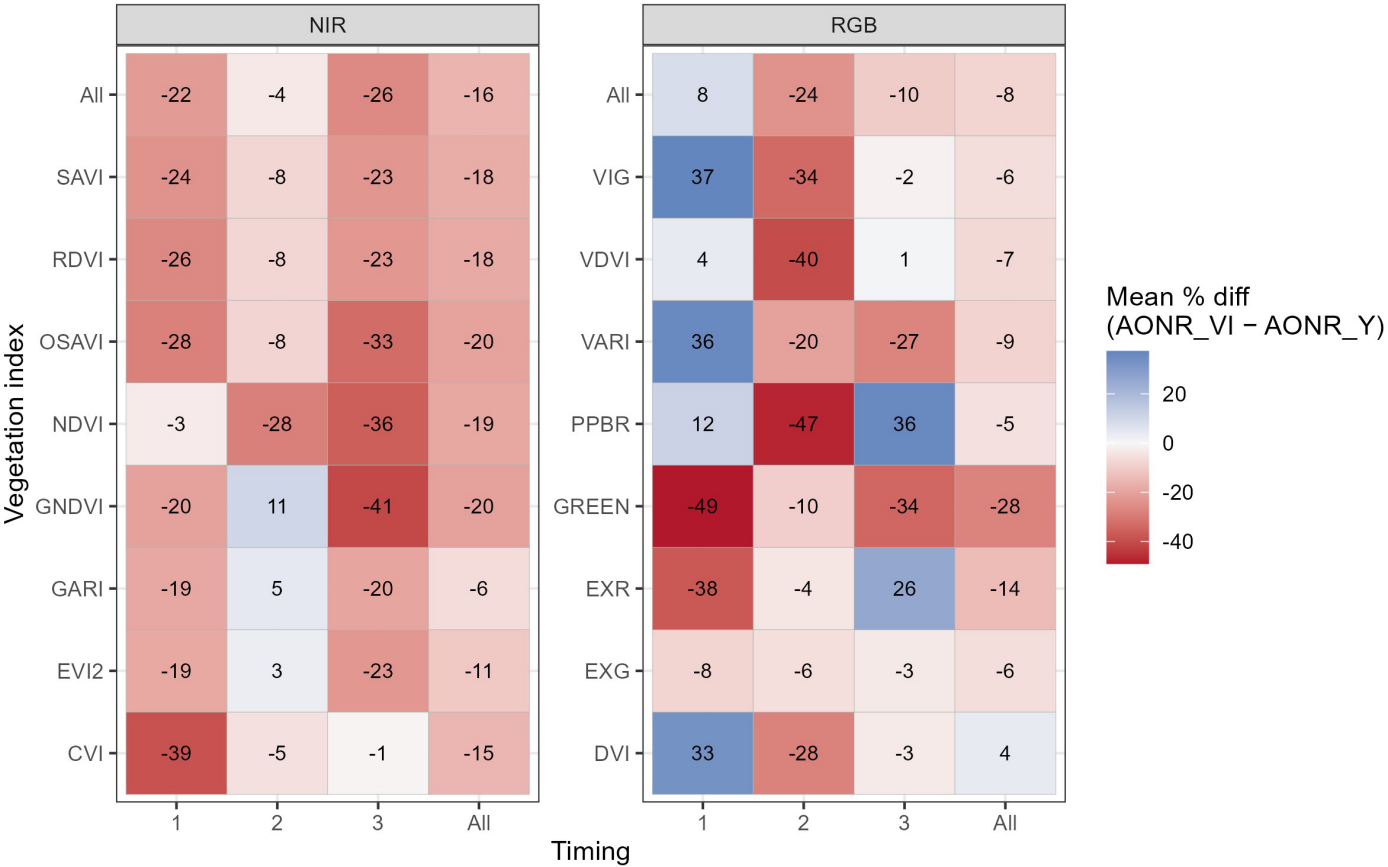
Percent differences between estimated (AONRvi) and observed AONR (AONRy). Values are shown by vegetation index (rows) and image timing (columns; 1, V7 and V8; 2, V10 and V11; 3, V15 and V16), including overall counts across timings and VIs (“All”). Panels are separated by VI type: near-infrared (NIR)-based indices (left) and RGB-based indices (right). Field names indicate tillage (ST, strip-till; CT, conventional tillage) and previous crop (C, corn; S, soybean). VIs names and formulas are described in Table 4.
